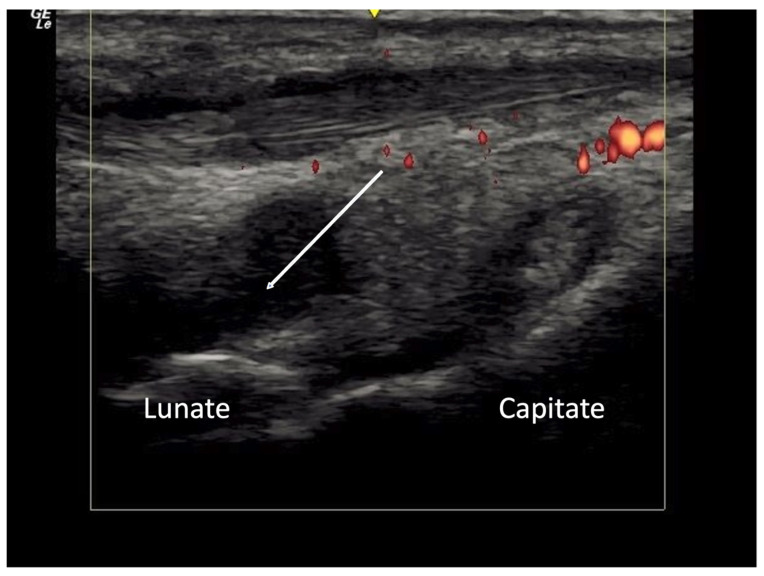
Longitudinal view of median dorsal wrist. Effusion (indicated by arrow) seen as anechoic, non-compressible material without Doppler signal within the radiocarpal joint.
